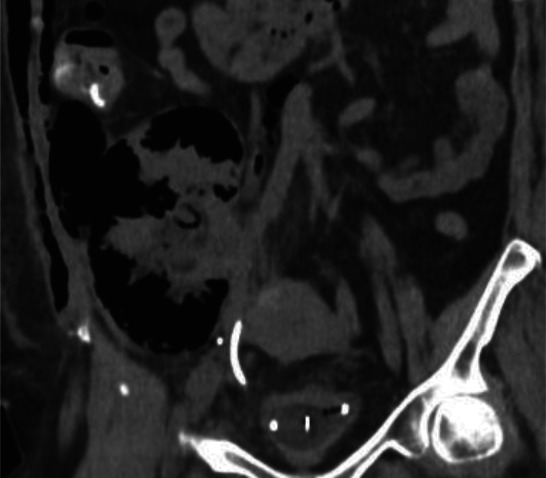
CT of the abdomen without contrast on initial presentation demonstrates emphysematous pyelonephritis within the transplanted kidney with intraperitoneal, right retroperitoneal free air, and subcutaneous emphysema along the right abdominal wall. CT, computed tomography.
